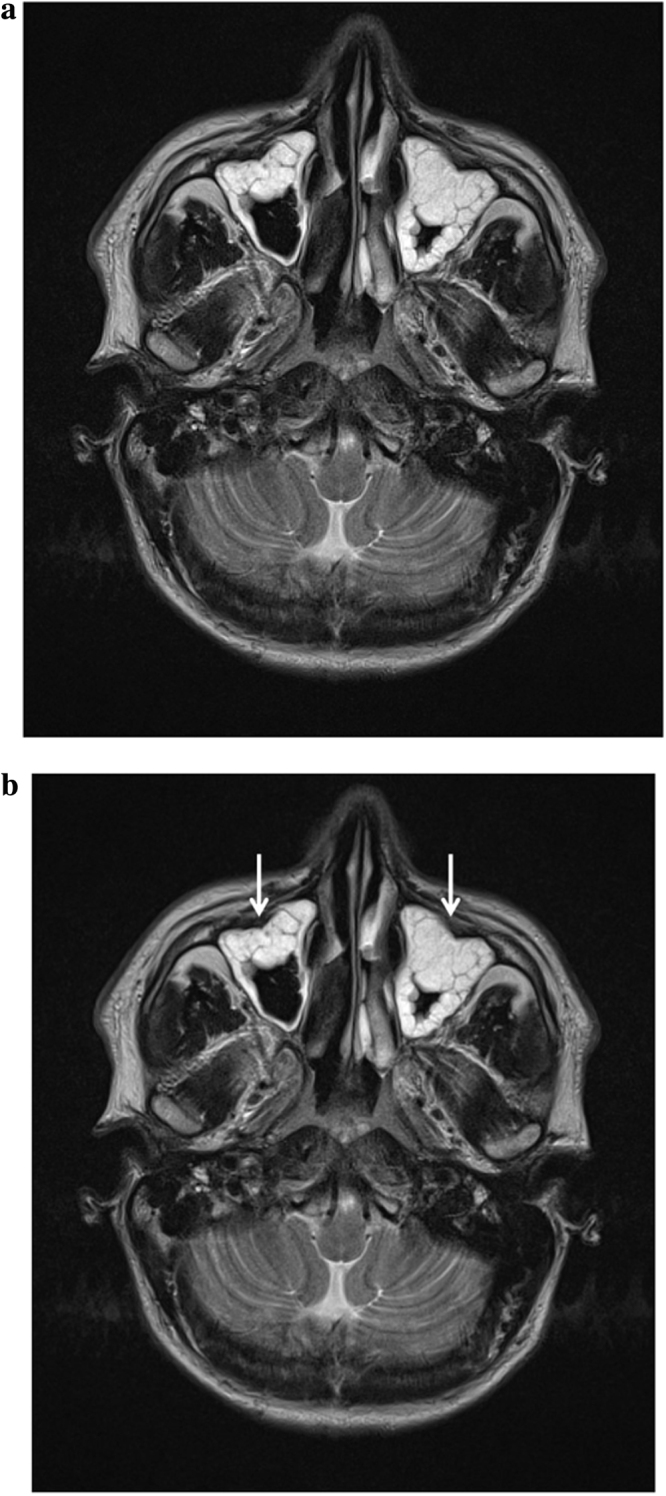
(a) MRI Head (T2 weighted) showing extensive loculated sinusitis (unmarked). (b) MRI Head (T2 weighted) showing extensive loculated sinusitis (unmarked).
